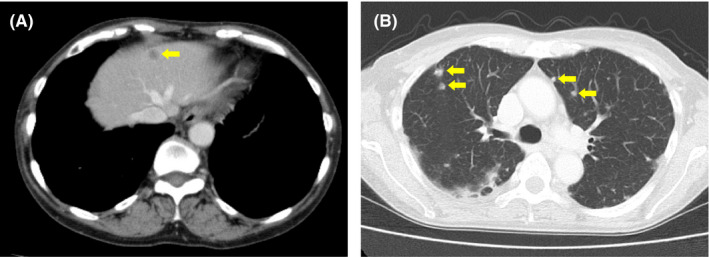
Computed tomography images taken at 5 months from initiation of radiotherapy. The arrows indicate metastatic tumors in the liver (A) and lung (B)
